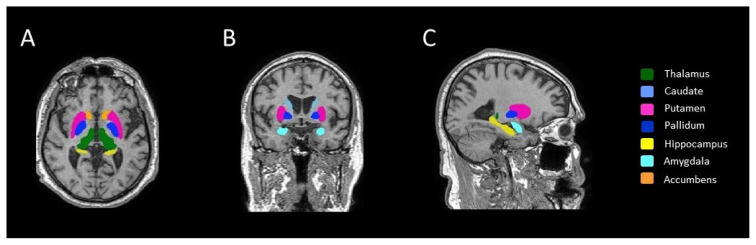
Example of automated segmentation of subcortical grey matter structure using FIRST on 3D T1-weighted images in A) axial, B) coronal, and C) sagittal planes.
